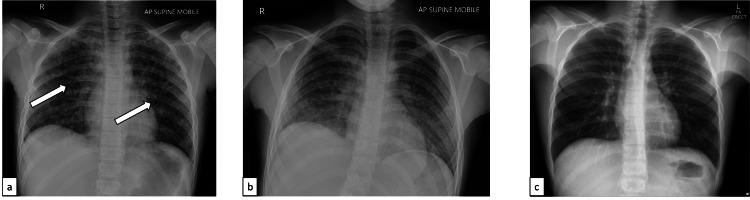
Patient's chest X-rays showing multiple small ill-defined nodular opacities noted in both lung fields. (a) Before IVIG (white arrows). (b) After IVIG. (c) After three months during follow-up IVIG: intravenous immunoglobulin
